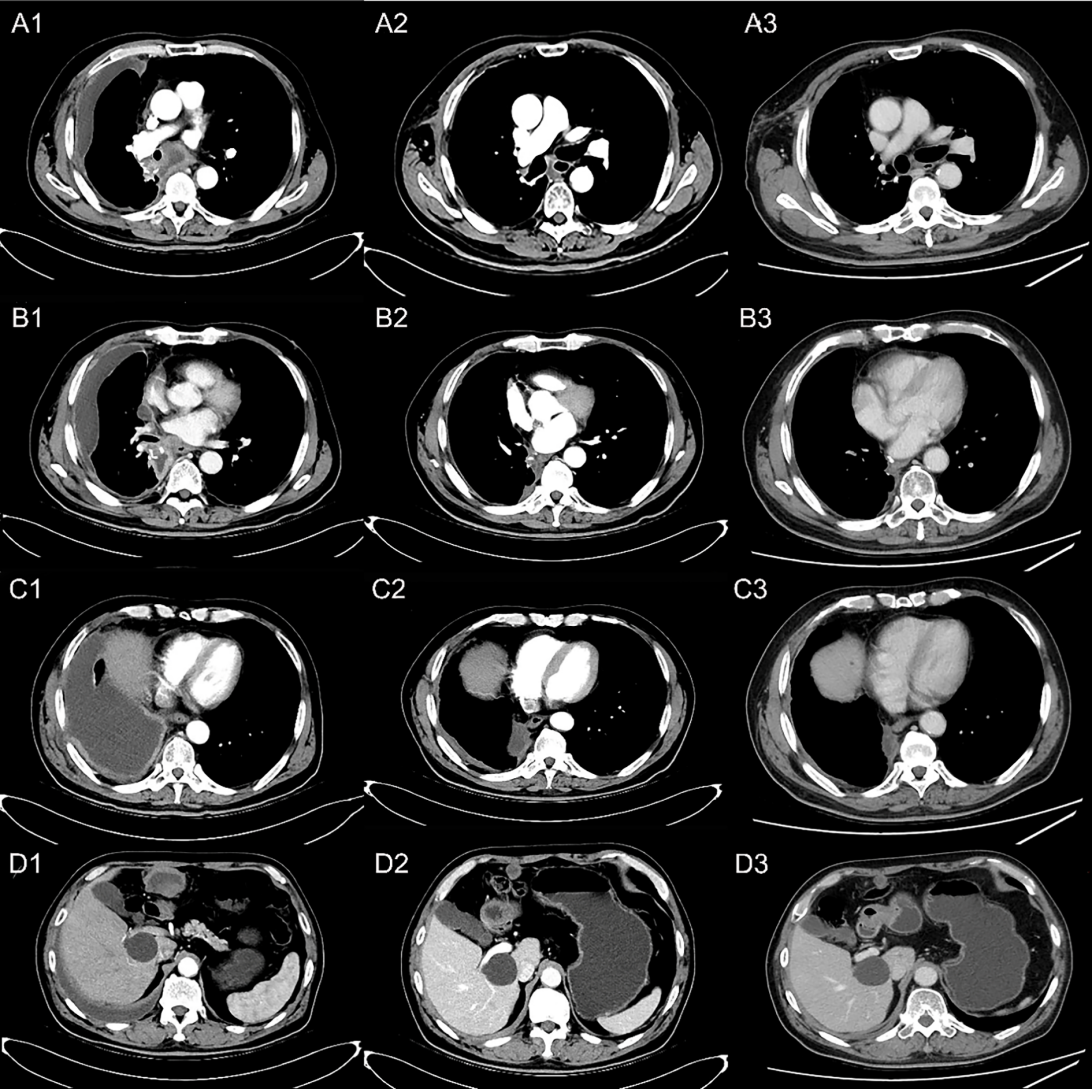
Reexamination results showing tumor regression by contrast-enhanced CT scan. (A) Occupation in mediastinum; (B) thickening of the right pleura; (C) soft tissue by the side of the right bronchial stump; (D) metastatic lesion on the right upper abdomen. (A1, B1, C1, D1) PD after one cycle of first-line chemotherapy; (A2, B2, C2, D2) disease PR after two cycles of the second-line treatment; (A3, B3, C3, D3) disease remaining as PR after four cycles of the second-line treatment.
